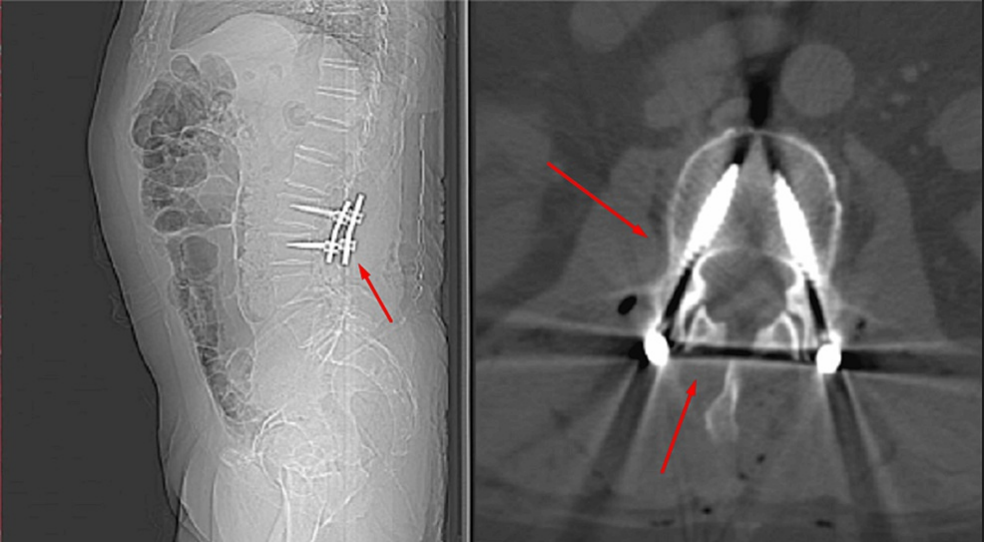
Postoperative radiography Radiography showed evidence of a successful stabilization of L2-L3 level with peduncle screws. No evidence of complications or pathological instability was seen
